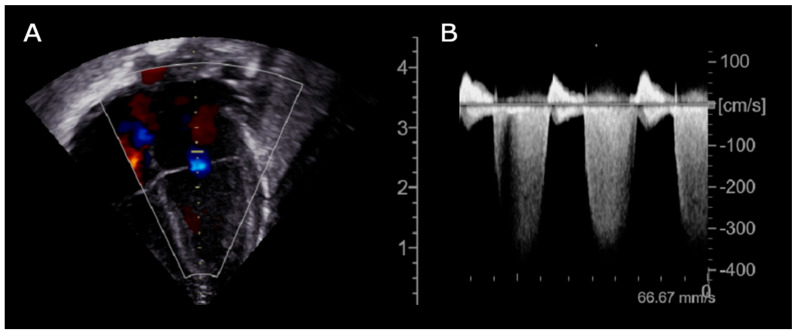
Echocardiogram (day +36), (A): Apical 4-chamber B-mode showing mitral regurgitation; (B): Continuous wave Doppler tracing of the mitral valve demonstrating a dense, holosystolic regurgitant jet with peak velocity exceeding 4 m/s. The complete systolic envelope and high signal intensity are indicative of significant mitral regurgitation with elevated left ventricular–left atrial pressure gradient.
